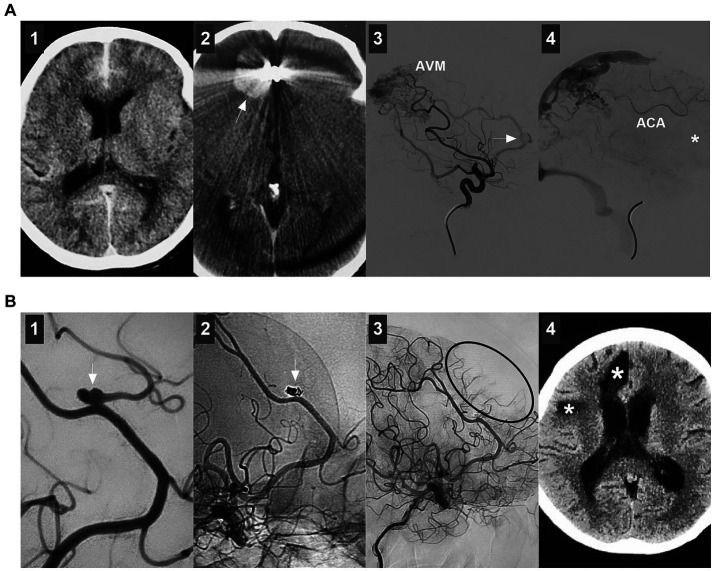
Complications of endovascular treatment for distal ACA aneurysms. (A) Panel 1: CT showing subarachnoid hemorrhage; Panel 2: Postoperative one-week CT showing a hematoma (arrow) in right frontal lobe; Panel 3: DSA showing that the flow-related aneurysm (arrow) on the ACA was incompletely coiled under the stenting assistance; Panel 4: DSA showing that the flow-related aneurysm (arrow) was incompletely coiled by parent artery occlusion at the asterisk location, the retrograde flow of ACA was sufficient. (B) Panel 1: DSA showing a distal ACA aneurysm (arrow); Panel 2: Unsubtracted DSA showing that the aneurysm (arrow) was coiled, and the branch from the aneurysm was occluded; Panel 3: DSA showing the insufficient blood flow by the occluded branch (circle); Panel 4: Six-month follow-up CT showing encephalomalacia (asterisks). ACA, anterior cerebral artery; AVM, arteriovenous malformation; CT, computed tomography; DSA, digital subtracted angiography.
